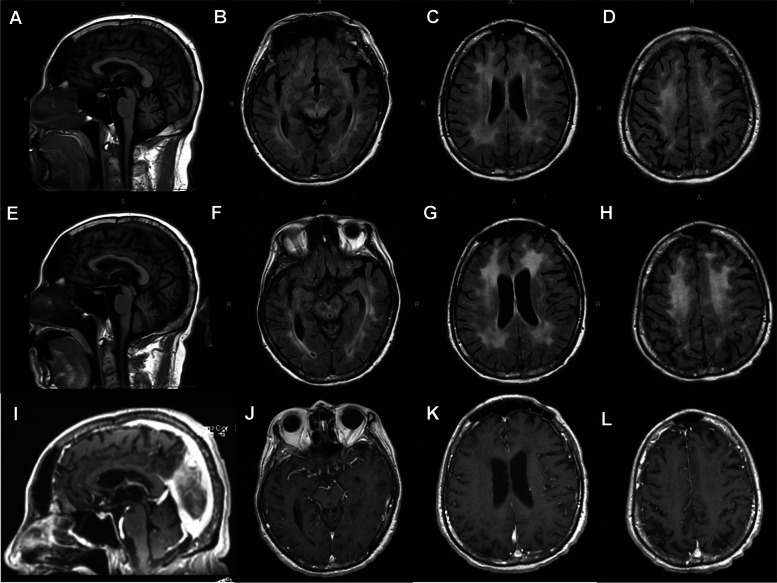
MRI Brain. T1-weighted sagittal without contrast (A, E), T2 FLAIR coronal (B-D, F–H), MPR of T1-weighted post-gadolinium sagittal (I) and T1- weighted post-gadolinium coronal (J-L) sections showing diffuse periventricular and deep white matter hyper-intensities with interval progression over 4 months (A-D vs. E-L). MRI: magnetic resonance imaging, FLAIR: fluid-attenuated inversion recovery, MPR: multiplanar reformation
